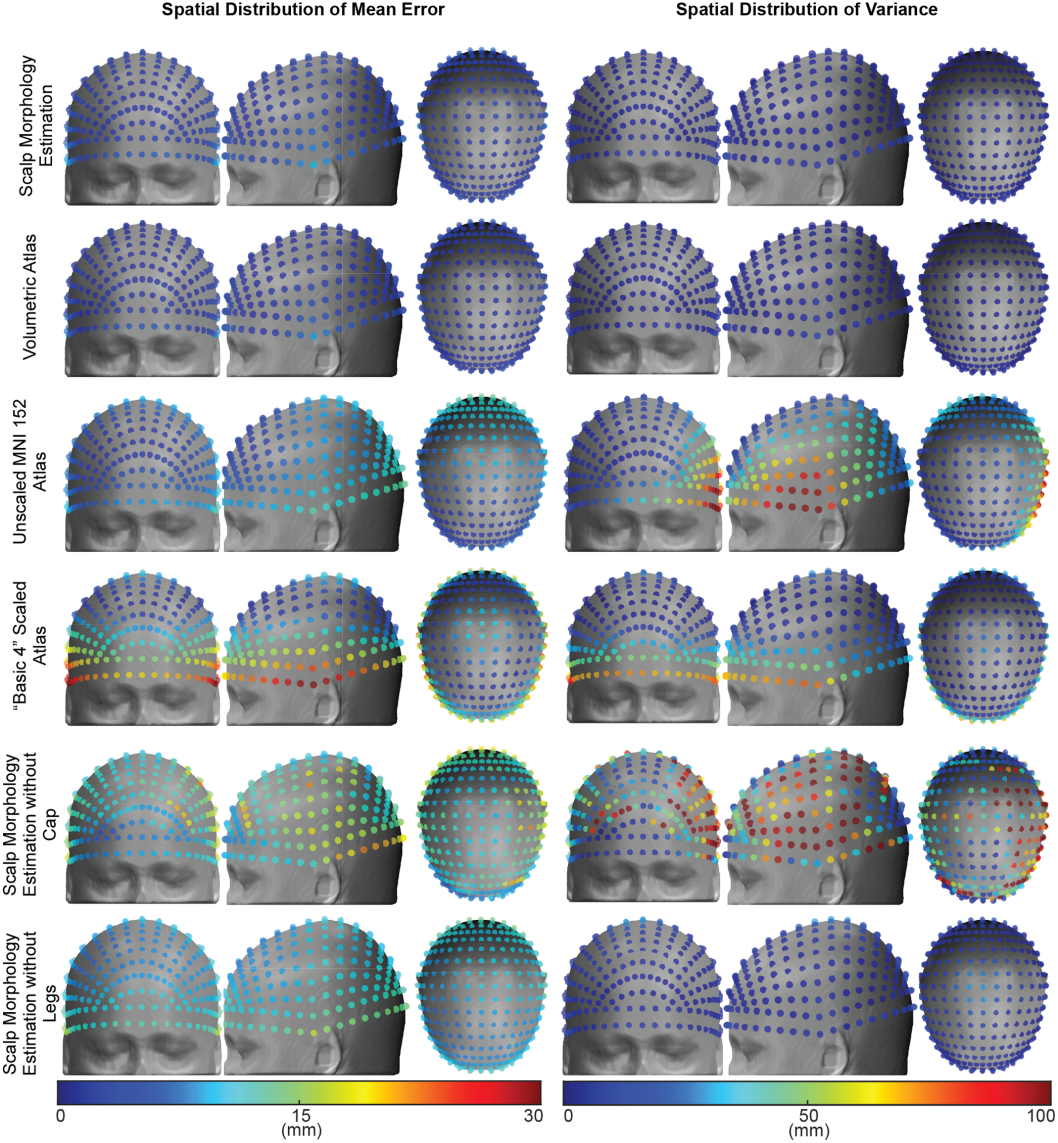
Spatial distribution of mean error and variance. The color of the sphere indicates the mean error (left) or variance (right) in millimeters at that location relative to the participant-specific MRI surface. Top to bottom: scalp morphology estimation, volumetric atlas, “basic 4” scaled atlas, unscaled atlas, scalp morphology estimation without photogrammetric cap, and scalp morphology estimation without photogrammetric module legs.
